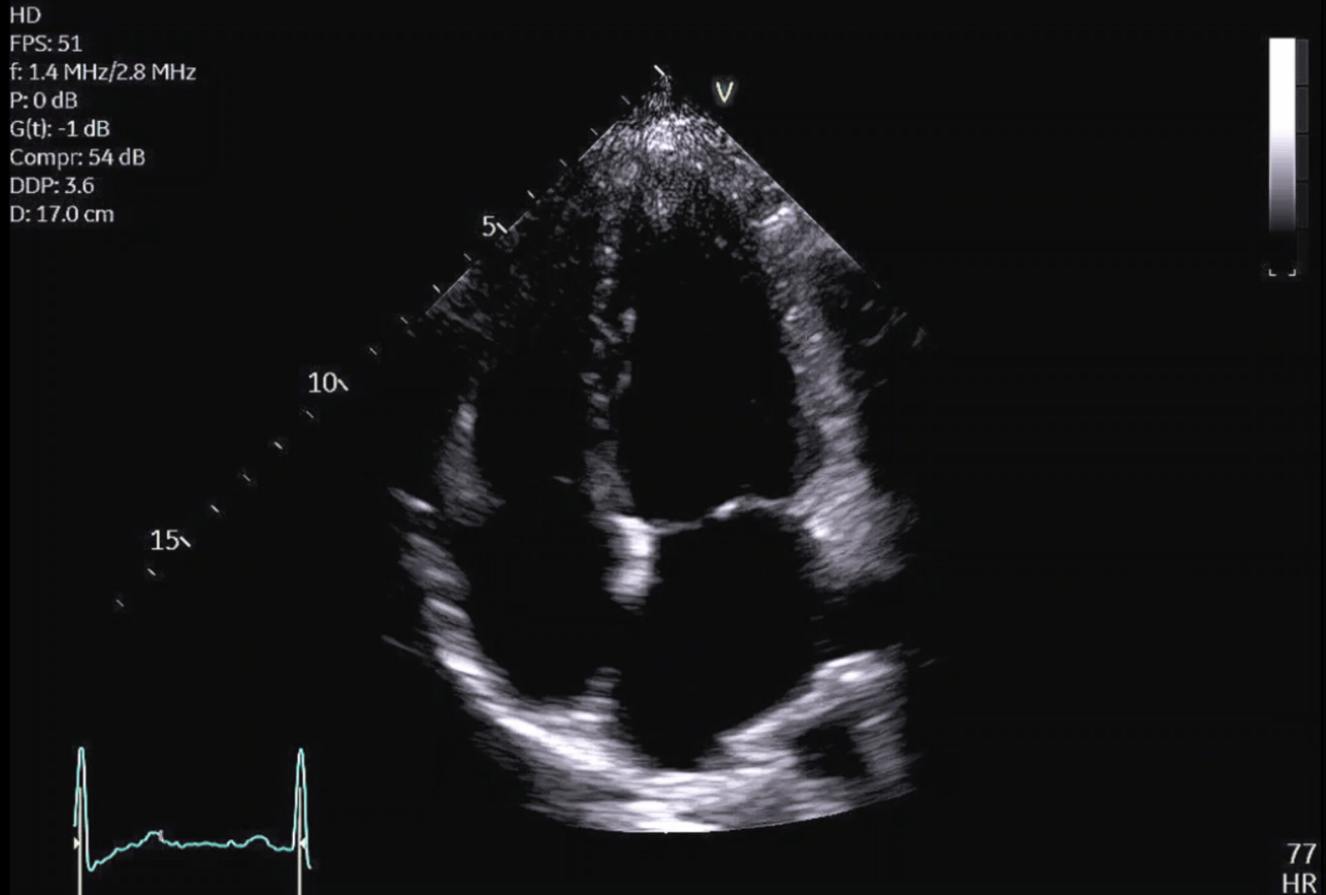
Transthoracic echocardiogram apical four-chamber view showing a structurally normal heart.
